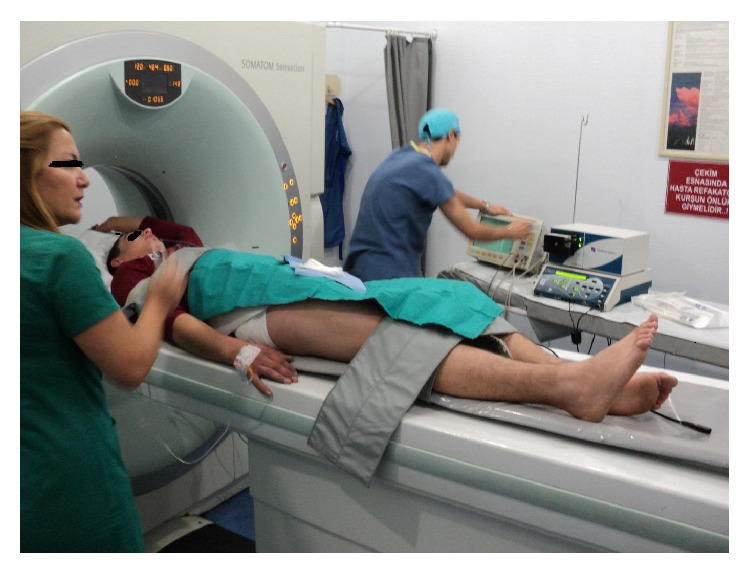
The patient was positioned on CT bed.
